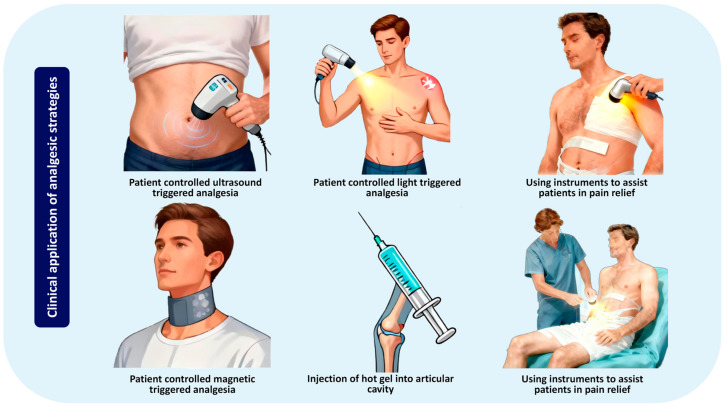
Applications of externally triggered local anesthetic nanoformulations. Ultrasound triggering. Stimulus: focused ultrasound waves from a transducer. Clinical Scenario: Ultrasound-guided nerve block for deep-seated nerves (e.g., transversus abdominis plane block). NIR light triggering. Stimulus: A beam of NIR light. Clinical Scenario: Patient-controlled, non-invasive analgesia for superficial nerves (e.g., post-operative shoulder pain). Clinical scenario: Non-invasive superficial nerve analgesia (such as postoperative shoulder pain) implemented by others to assist patients. Magnetic triggering. Stimulus: Static magnetic field for targeting; alternating magnetic field (AMF) for hyperthermia-triggered release. Clinical scenario: Spatial targeting and on-demand release for complex anatomical regions (e.g., brachial plexus, neck thyroid surgery). Thermal triggering. Stimulus: Ambient body heat or an external mild heat source. Clinical scenario: Injectable depot for prolonged analgesia at routine surgical sites (e.g., Hip and knee joint). Assisting patients with abdominal pain relief by others. The authors created this figure using WPS Presentation (12.1.0.24657).
